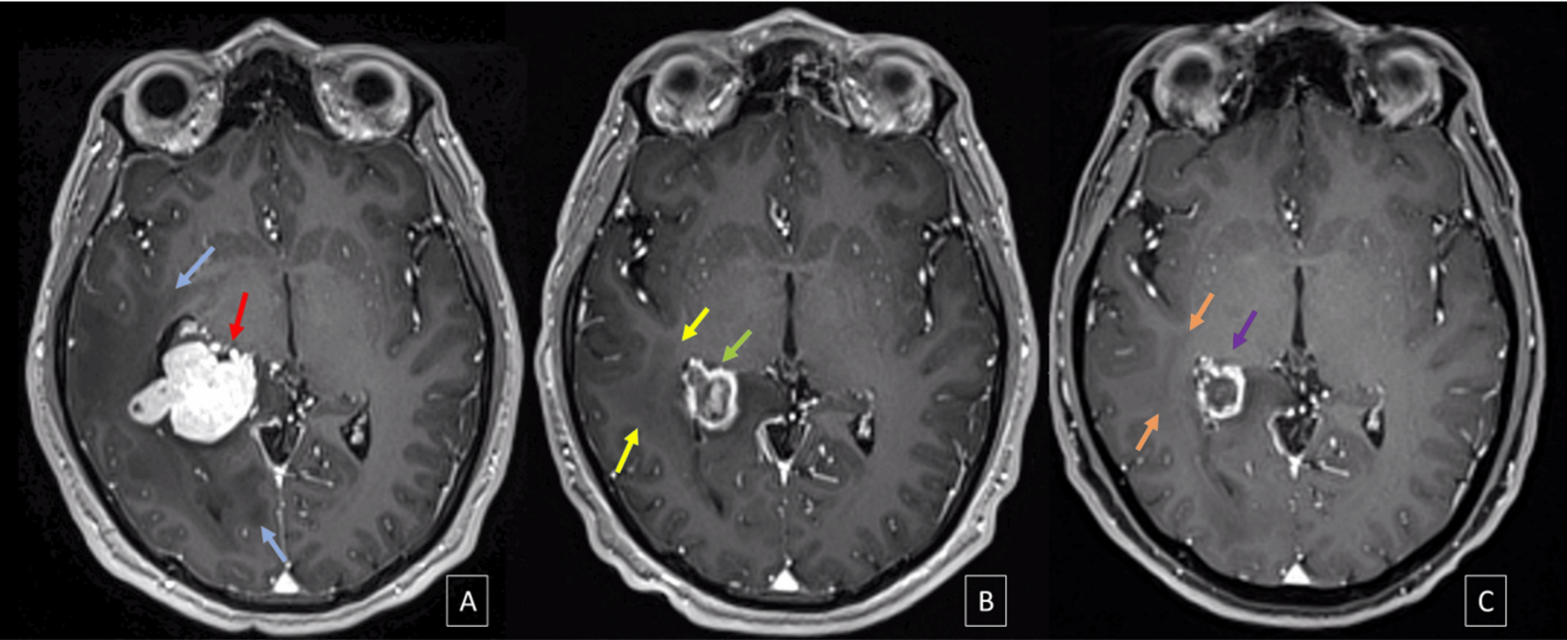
3D axial T1 MPRAGE G+ of a 41-year-old male patient with brain metastasis from clear cell renal carcinoma MPRAGE: Magnetization prepared-rapid gradient echo; MRI: magnetic resonance imaging; SRS: stereotactic radiosurgery In the baseline MRI (A), prior to SRS, a predominantly solid lesion with a homogeneous enhancement pattern is observed (red arrow), along with associated perilesional edema (blue arrows). In MRI-2 (B) at three months, there is a reduction in lesion size, with a decrease in the solid component, trending toward a cystic form due to progressive intralesional cavitation and the development of central necrosis (green arrow), as well as a decrease in perilesional edema (yellow arrows). In MRI-3 (C) at six months, further reduction in perilesional edema is seen (orange arrows), along with a decrease in lesion size, with a ring enhancement pattern, areas of peripheral thickening, and an increased central necrotic component (purple arrow)
